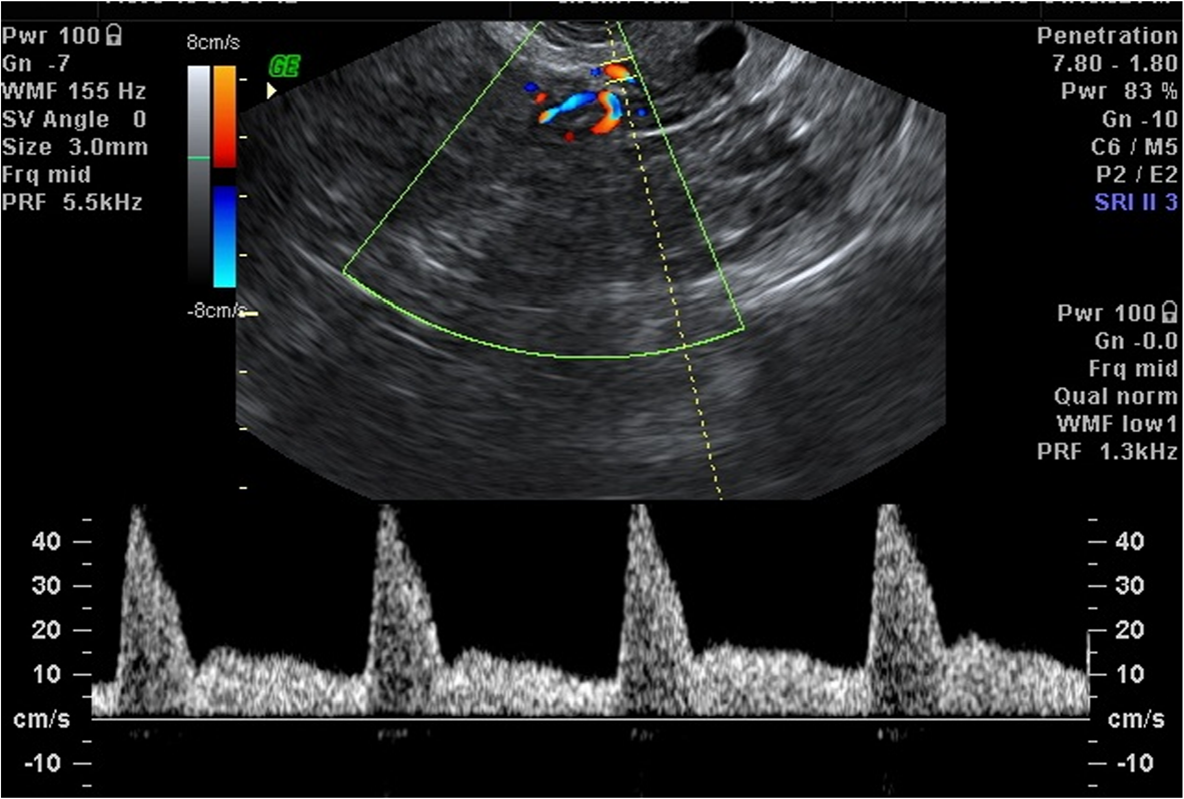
Two-dimensional color Doppler of uterine artery flow velocity waveforms
